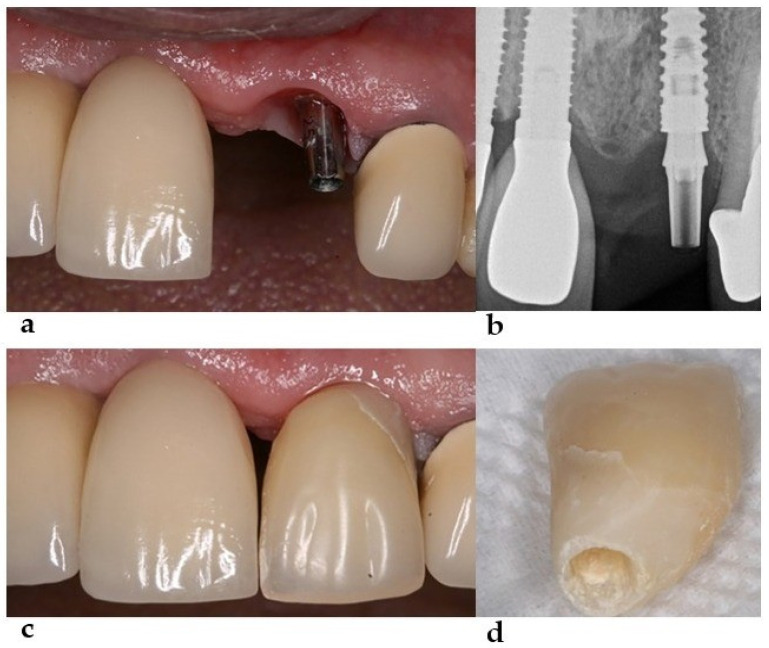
(a) Universal abutment installed over the implant, displaying the implant inclination. (b) Radiographic aspect of the installed abutment. (c) Provisional restoration over the abutment. (d) Provisional restoration showing a low thickness at the distal region.
